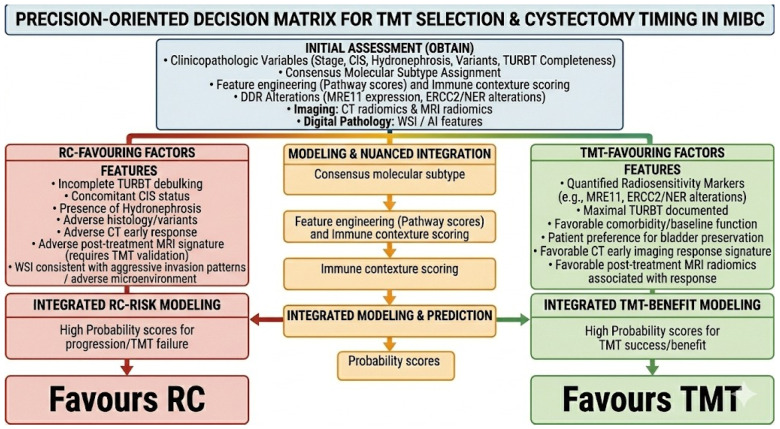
Precision-Oriented Decision Matrix for TMT Selection & Cystectomy Timing in MIBC. (MIBC: muscle-invasive bladder cancer; TMT: trimodality therapy; RC: radical cystectomy; CIS: carcinoma in situ; TURBT: transurethral resection of bladder tumor; DDR: DNA damage response; NER: nucleotide excision repair; WSI: whole-slide imaging; AI: artificial intelligence; CT: computed tomography; MRI: magnetic resonance imaging; pCR: pathological complete response; AUC: area under the curve.)
